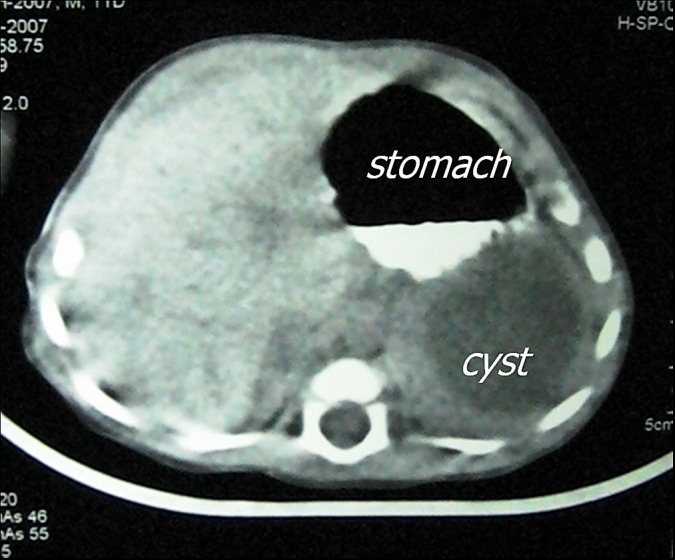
Figure 2: Abdominal CT showing the relation between the cyst and stomach.
